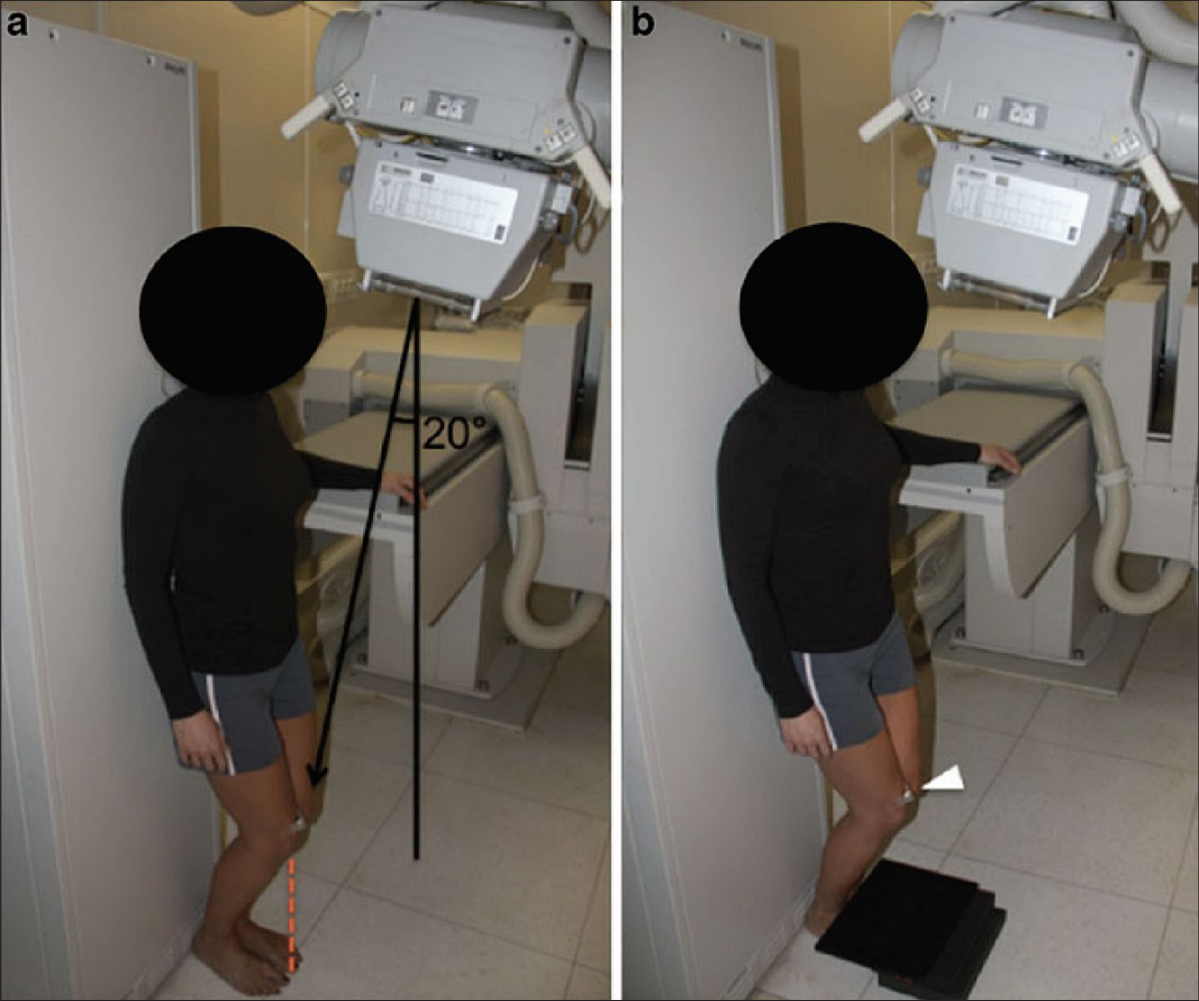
Standing *skyline* radiograph. (a) The subject stands against a flat surface with the back, hip, and heel in a coplanar position. The anterior aspect of the patella is beneath the tip of the corresponding big toe (dashed red line). The X-ray beam is angled 20° toward the patient and centred on a point about 2 cm posterior to the anterior aspect of the patella. (b) The cassette is positioned parallel to the floor on a piece of foam rubber, which adapts itself to the underlying feet. A 25-mm iron ball (white arrowhead) was positioned at the anterior aspect of the right knee in order to correct for magnification [18].
